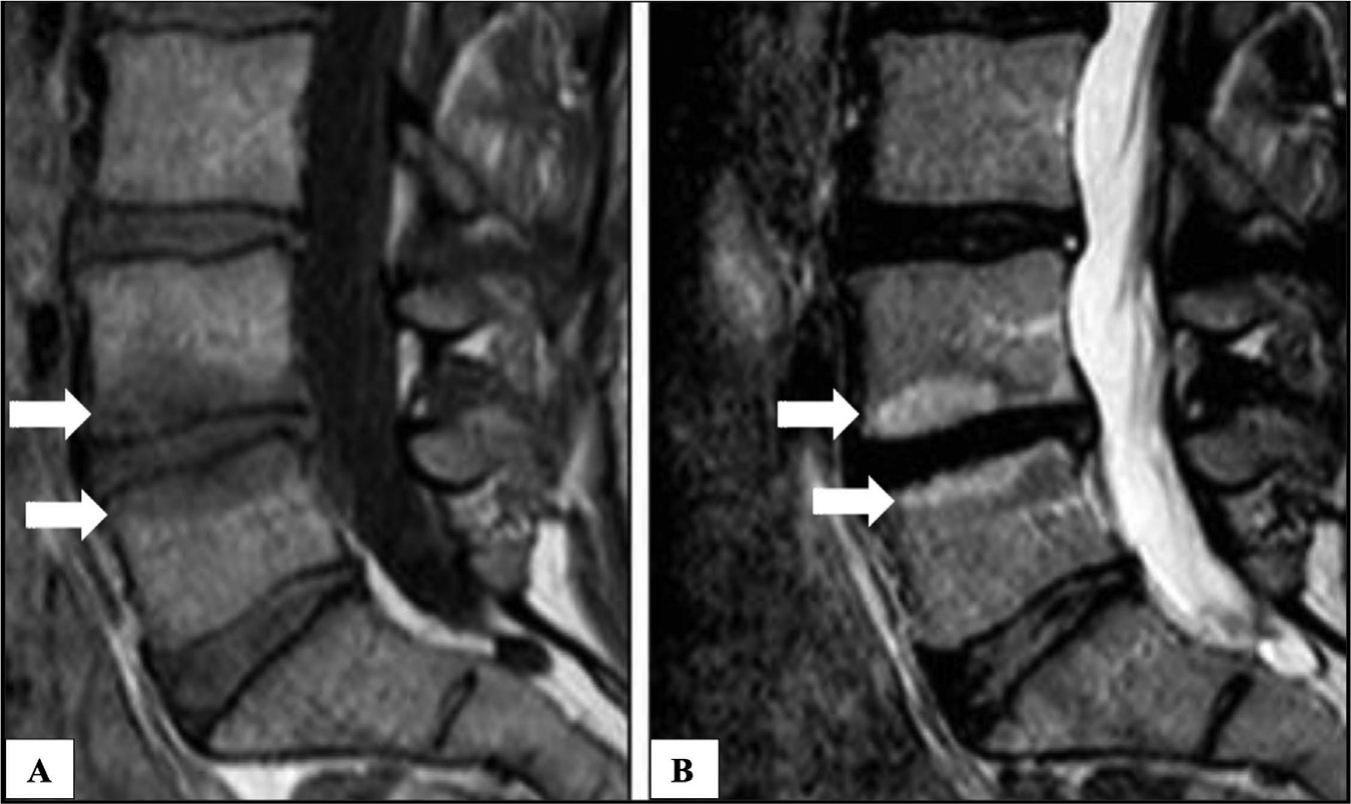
Type 1 Modic changes as visualized on magnetic resonance imaging. Sagittal T1-weighted (A) and T2-weighted (B) images of the lumbar spine showing hypointense signal on T1 and hyperintense signal on T2, indicative of bone marrow edema and inflammation at the vertebral endplates. These changes suggest an early degenerative process in the intervertebral disc and adjacent vertebral bodies. White arrows identifying bone marrow edema and micro-fractures around the L4-L5 intervertebral space. (Image adapted from Neurosurgery Education Wiki: Modic type 1 changes (https://neurosurgery.education/wiki/doku.php?id=modic_type_i_changes), Licensed under Creative Commons Attribution-ShareAlike 4.0 International Deed).
